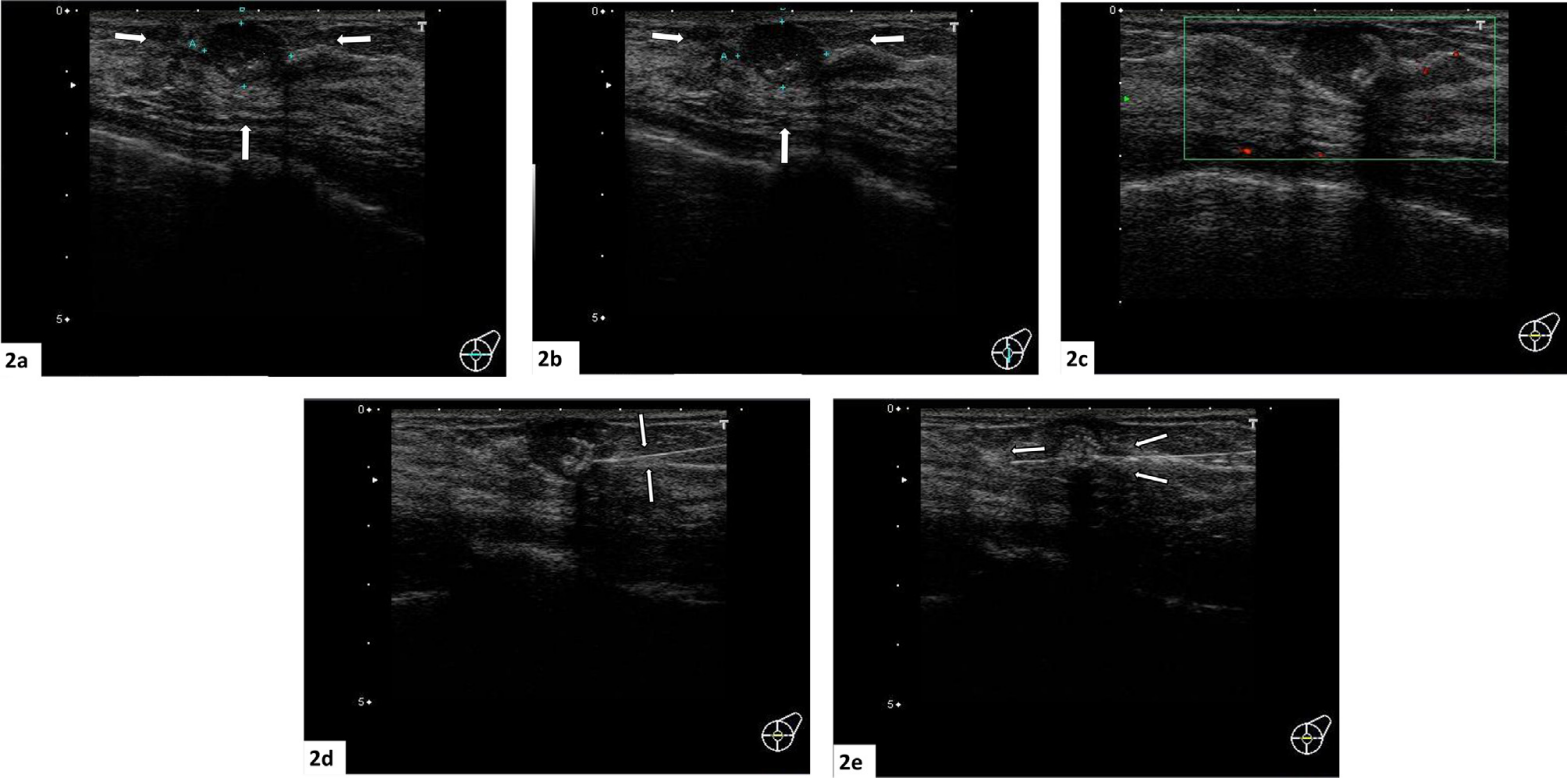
Breast ultrasonography; 2(a,b,c). Ultrasonography revealed a poorly demarcated tumor measuring 14.3 × 14.8 × 10.7 mm with relatively smooth margins and inhomogeneous high-echo content (Fig. 2a and b). Color Doppler US showed a hypovascular pattern (Fig. 2c). (For interpretation of the references to colour in this figure legend, the reader is referred to the Web version of this article.)
